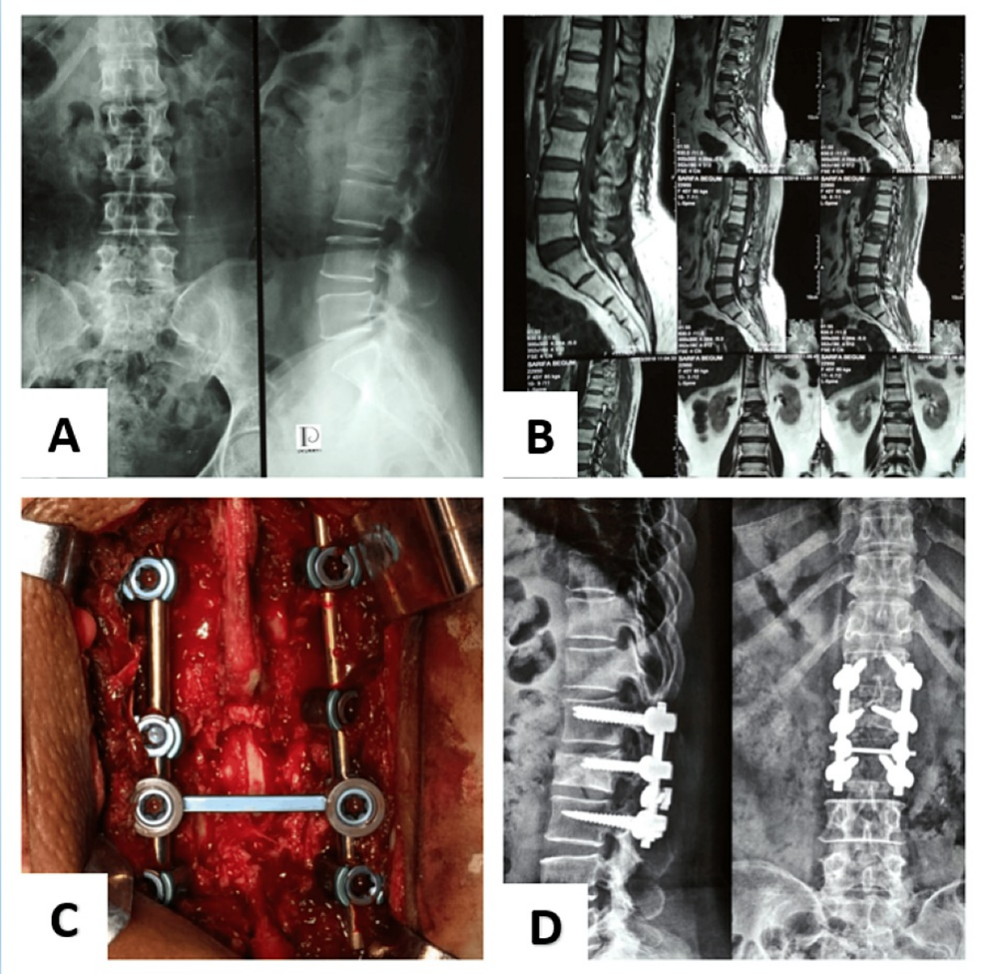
Short-segment fixation including fracture level. A. Preoperative X-ray of the lumbosacral spine anteroposterior and lateral view. B. T2-weighted sagittal section (MRI of the lumbosacral spine) showing burst # L2. C. Peroperative image showing short-segment fixation including fracture level with cross-link. D. X-ray of the lumbosacral spine anteroposterior and lateral view at the final follow-up.
